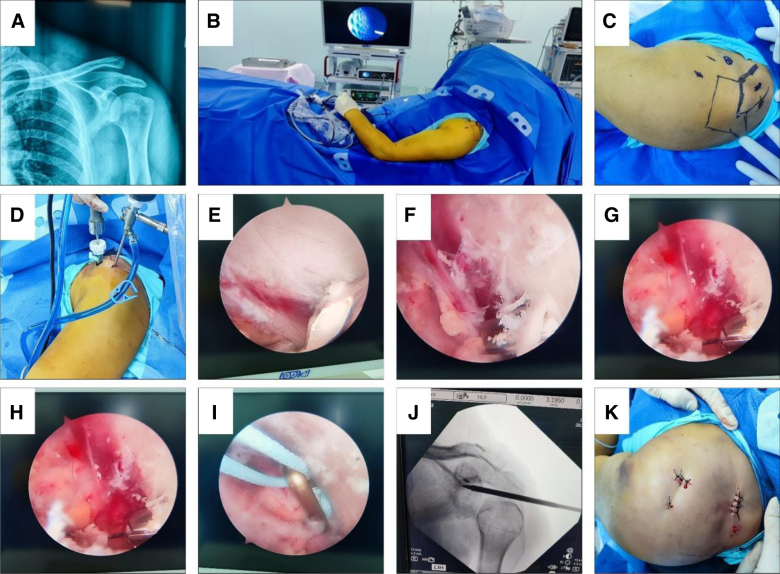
(A) X-ray of a 49-year-old male patient with left acromioclavicular joint dislocation before surgery. (B) Patient position for shoulder arthroscopy. (C) Body markings and access position. (D) Establishment of anterior and anterolateral channels. (E) Placement of cruciate ligament locator. (F) Drilling of the channel with a 4.5 mm cannulated drill. (G) Insertion of a nickel wire guide wire along the cannulated drill. (H) A traction wire with a loop plate at the tail end of the nickel wire. (I) The traction loop plate passes through the base of the coracoid process. (J) Intraoperative fluoroscopy to evaluate the reduction quality. (K) Postoperative appearance of the wound.
